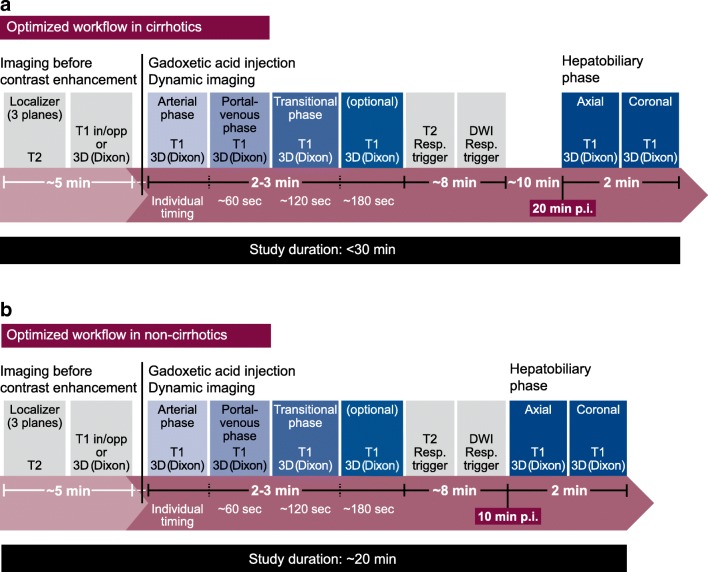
Optimised MRI protocol using gadoxetic acid. DWI, diffusion-weighted imaging
